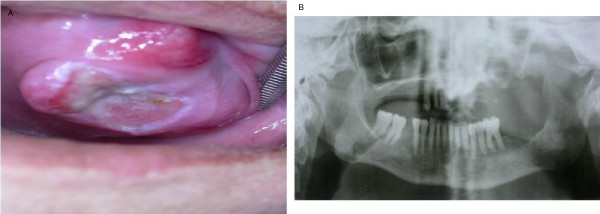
(a) Intra-oral view showing the clinical aspect of case 3. (b) Panoramic radiograph showing the destruction of bone with extensive indefinite borders in the maxillary molar region of case 3.
